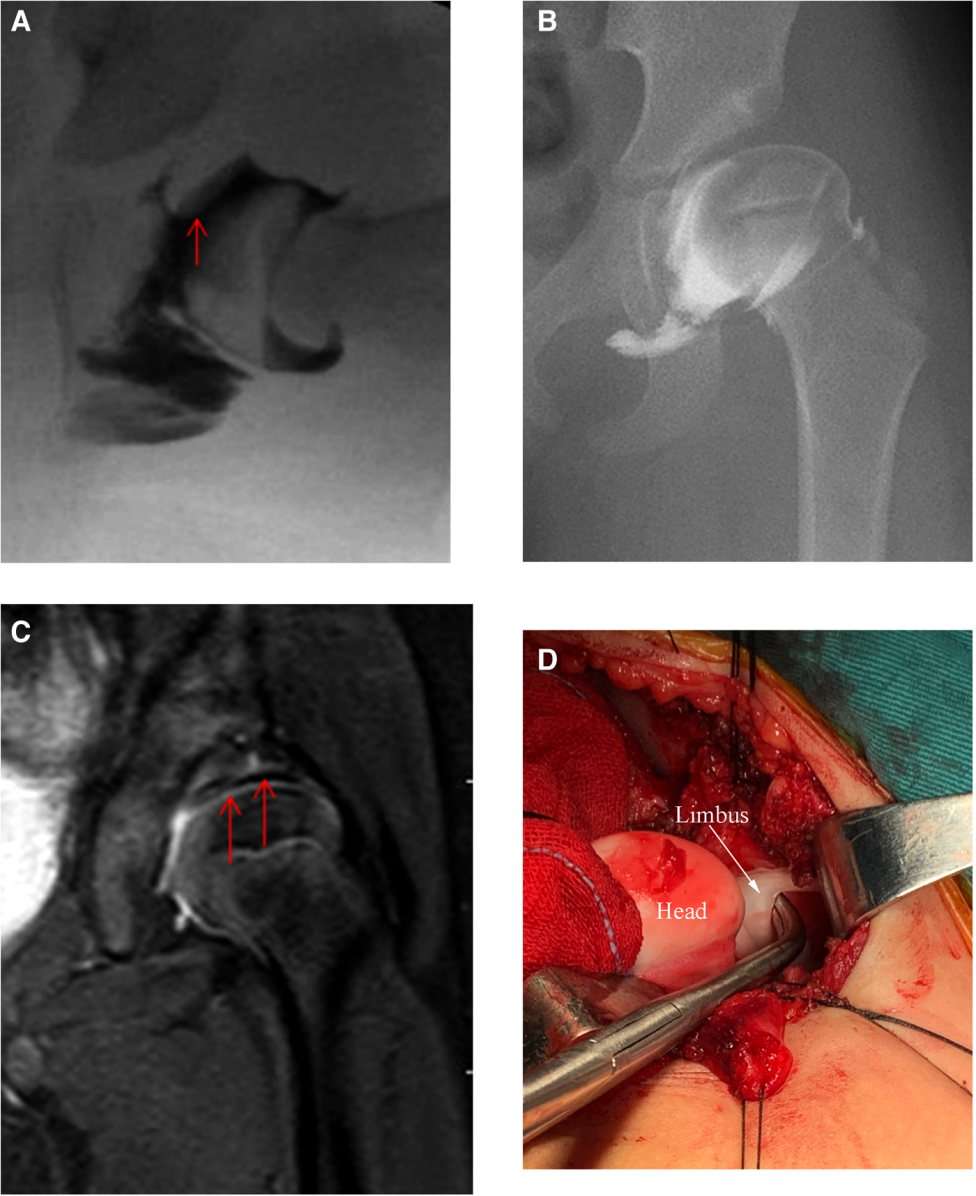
A 14-month-old female patient who underwent closed reduction of left hip dislocation. (A) Arthrography showed hypertrophic inverted limbus tissue during reduction; (B) Residual acetabular dysplasia after reduction for 3 years. Arthrography showed no abnormal tissue between the femoral head and acetabulum; (C) Coronal MRI showed a thin layer of hypointense tissue embedded in the superior joint space (arrows); (D) Intraoperatively confirmed limbus inversion.
